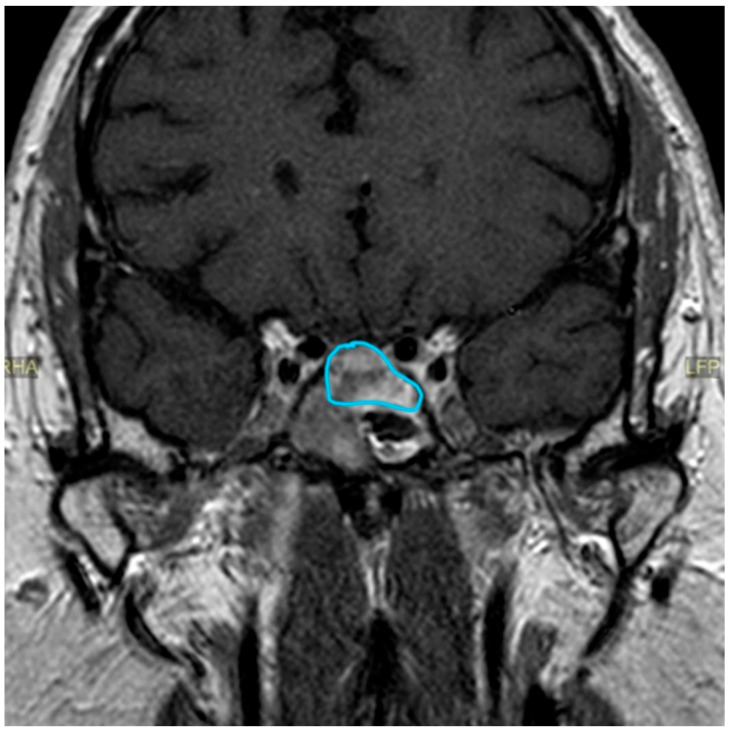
MRI, June 2021. The adenomatous rest reduced its volume, particularly in its cranial part, so it was no longer in contact with the gyrus rectus and the overlying subcallosal area. MRI, magnetic resonance imaging.
